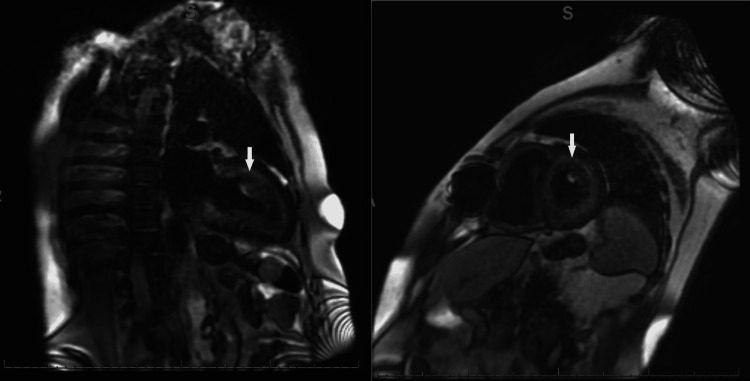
(Left, right) Gadolinium-enhanced cardiac MRI showing diffuse heterogeneous enhancement overlying the entire subendocardial circumference extending into at least 50% of the myocardial thickness (white arrows).
